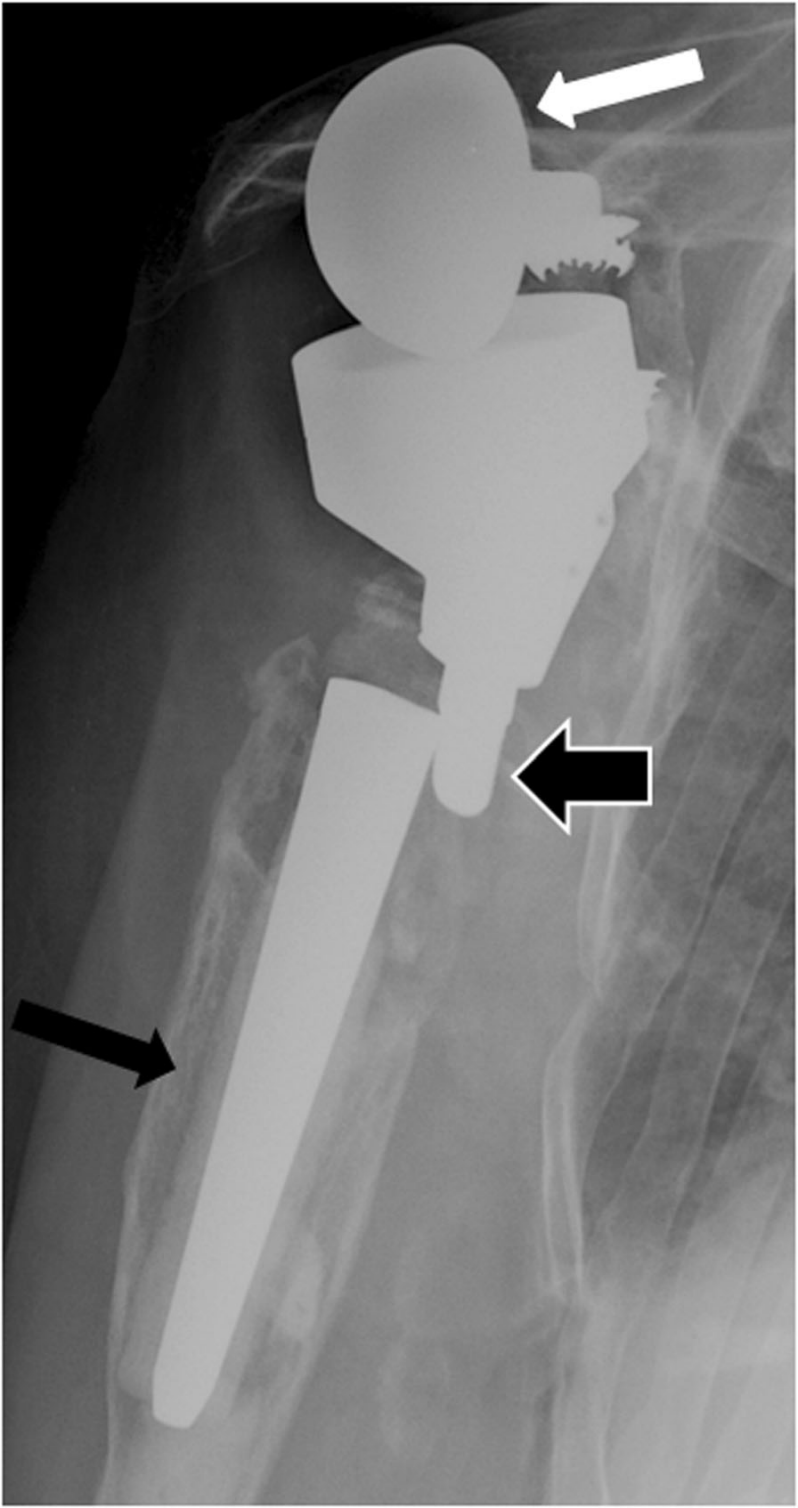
Anteroposterior radiograph of a reverse shoulder arthroplasty in a 73-year-old patient shows humeral implant disjunction with unscrewing of the two components (thick arrow). This is secondary to both humeral loosening resulting in chronic abnormal mobility of the implant (black thin arrow), and glenoid loosening with the glenoid component “wedged” under the acromion (white thin arrow)
